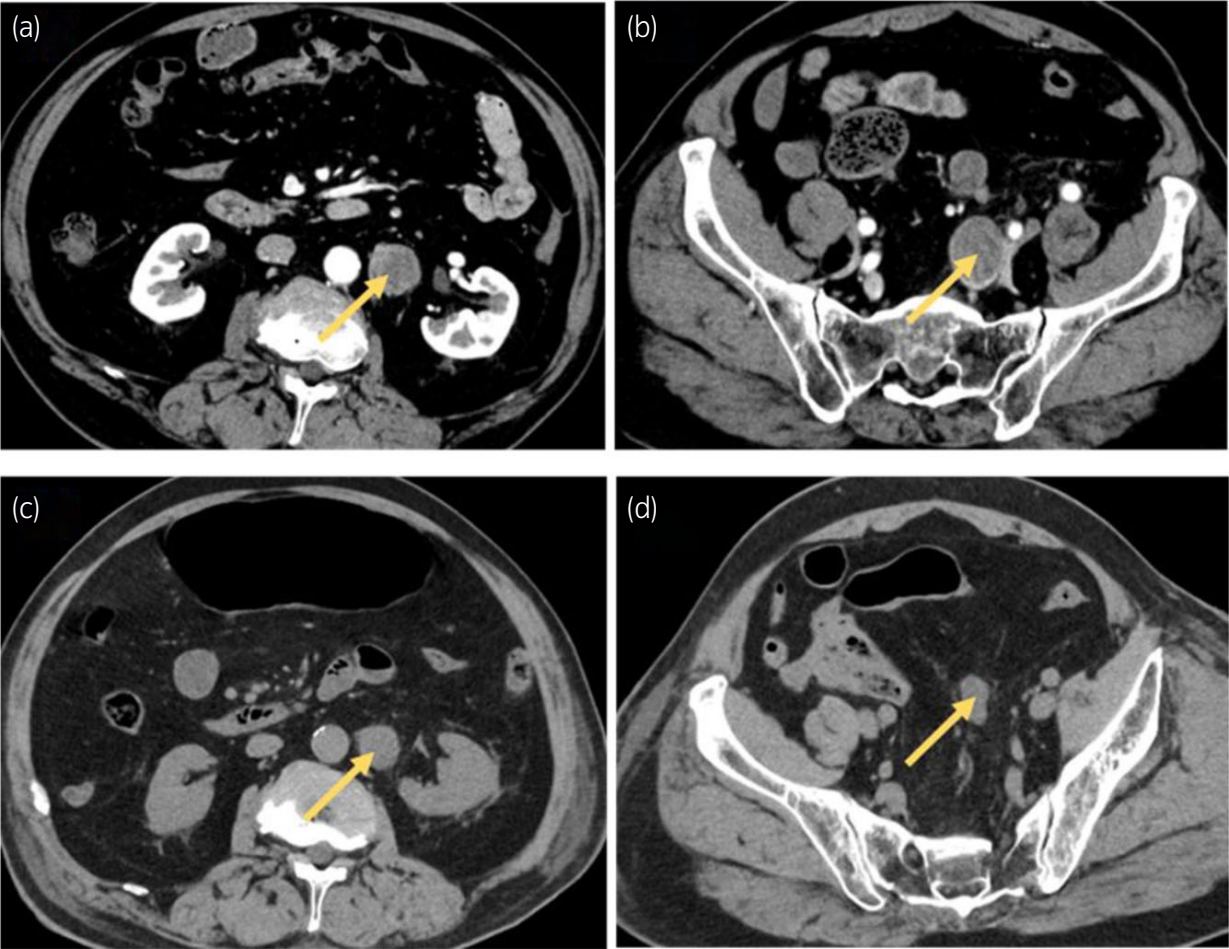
Abdominal CT. (a, b) Contrast‐enhanced CT retroperitoneal and intrapelvic lymph node metastases before EV administration (arrows). (c, d) Plain CT lymph node metastases (arrows) are slightly reduced 16 days after the start of EV administration.
